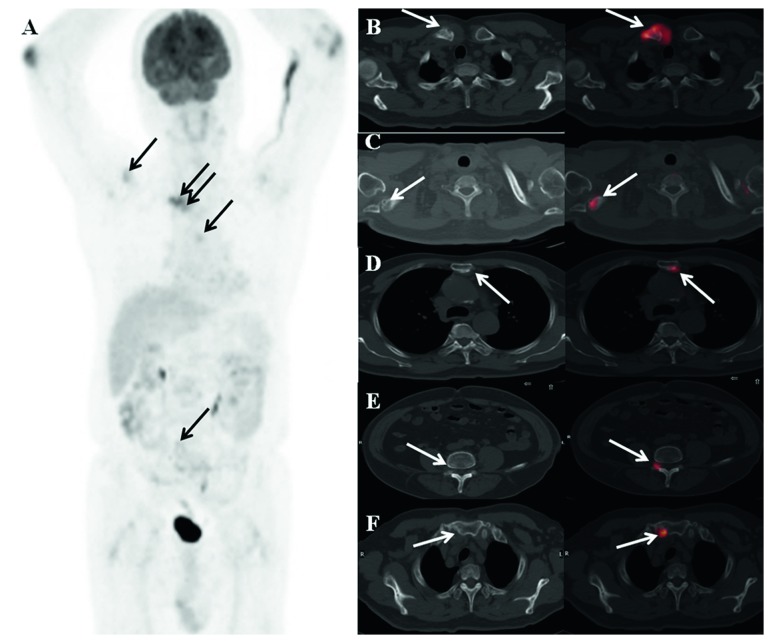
^18^F-FDG PET/CT imaging in a 72-year-old man with MM: (a) MIP image; (b–f) transaxial CT and fused images. In this patient, the descriptive criteria (IMPeTUs) were BM(3), F3.SP.ExtraSP(4), L2, PM, EM.N(2), where BM3 indicates that bone marrow uptake is <liver but >mediastinum, F3 indicates 4 to 10 lesions (arrows), SP indicates the spine, ExtraSP indicates outside the spine with (4) indicating reference lesion uptake >liver uptake + 10%, L2 indicates that 1 to 3 lesions were also lytic, PM indicates a bone lesion in surrounding soft tissues with bone cortical interruption, EM indicates at least one extramedullary lesion, and N indicates that the extramedullary lesion was nodal disease with (2) indicating extramedullary lesion uptake ≤mediastinum. This patient was classified as IIA according to DS Plus.
